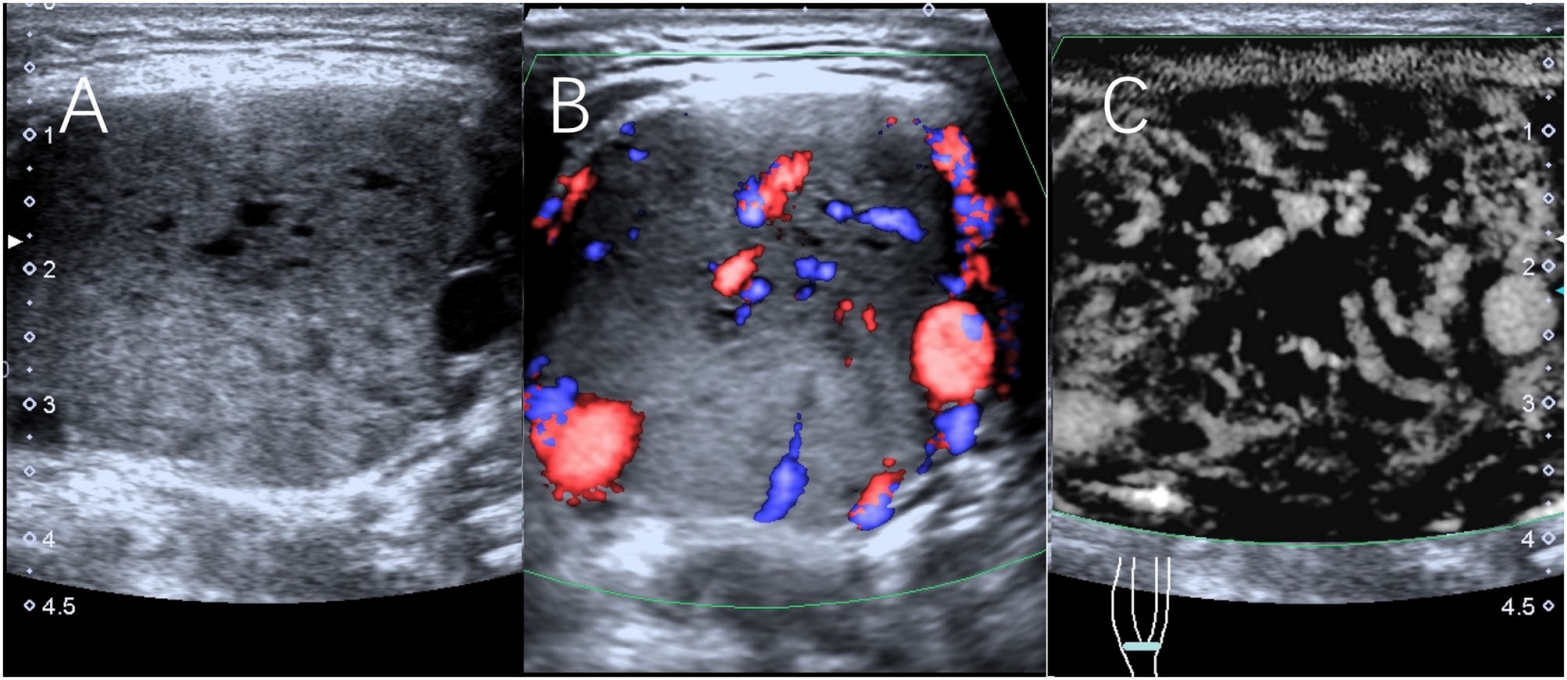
Scans from a 32-year-old man with a right CBT lesion measuring 4.4 cm. (A) Grayscale sonography showing the lesion. (B) The lesion was classified as having Adler II vascularity on CDFI. (C) The lesion was classified as having Adler III vascularity on SMI.
